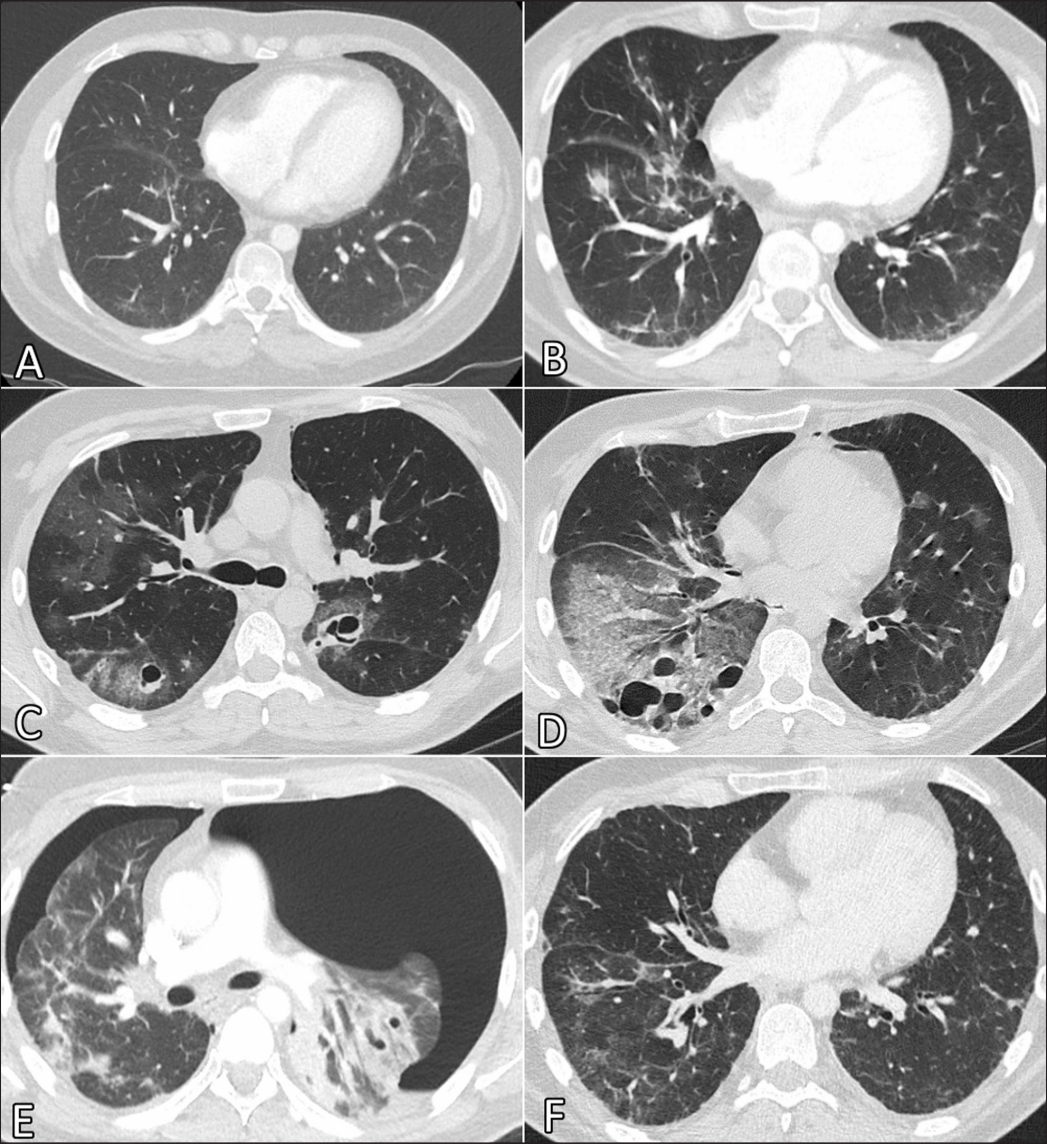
Progression of pulmonary abnormalities on chest CT.
